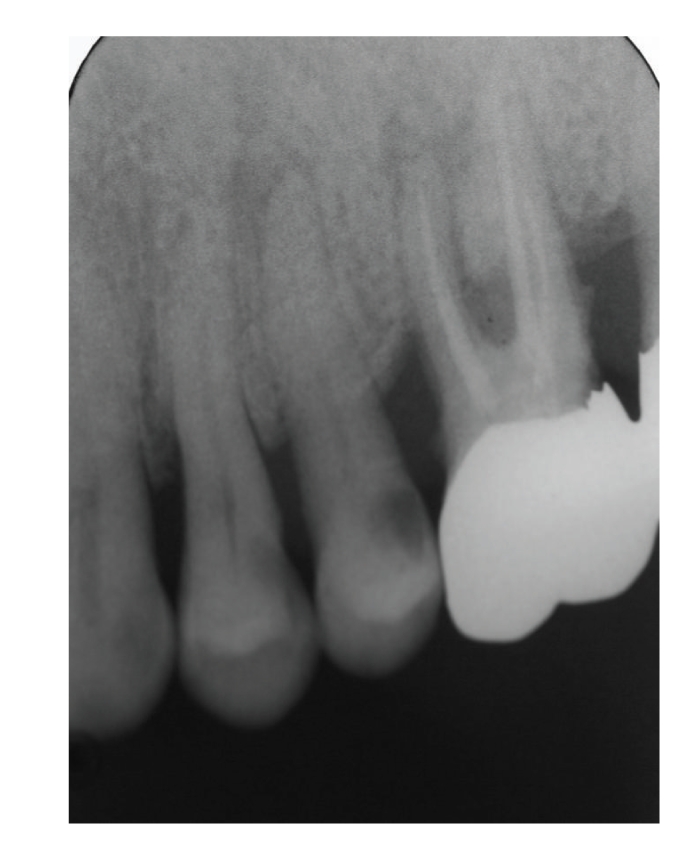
Case 1: Radiography at the first visit showing radiolucency at the apex area of tooth 46.
